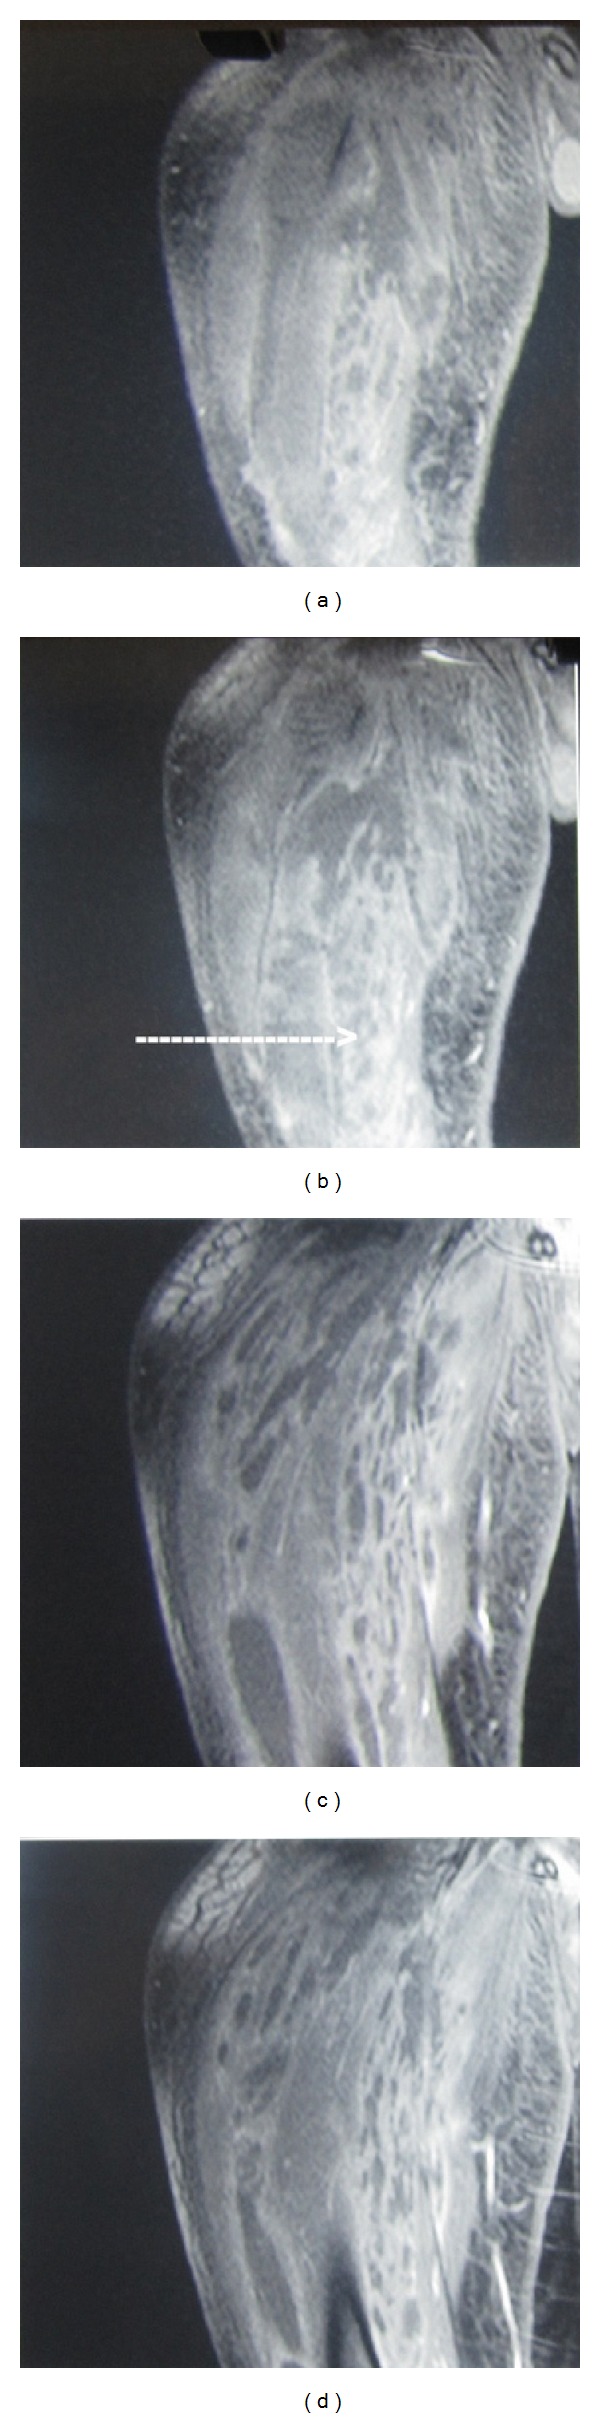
MRI right thigh—MRI coronal T1W—showing a diffuse septated intramuscular collection suggestive of pyomyositis of quadriceps femoris.
